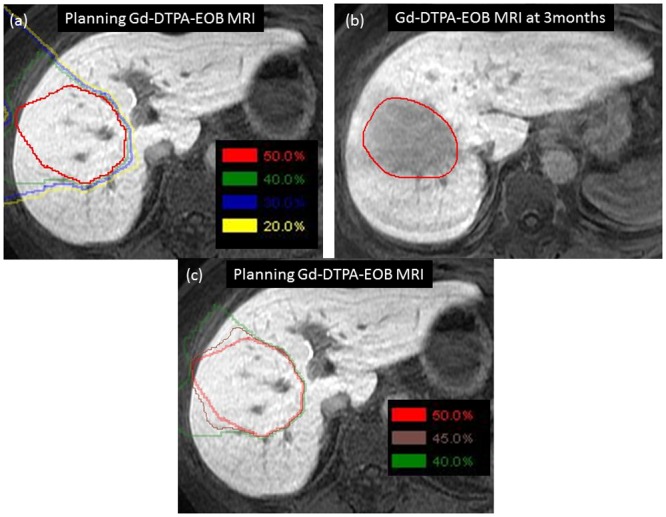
Method for defining the TD for FLR using hepatobiliary phase Gd-EOB-DTPA MRI at 3 months after PBT. The isodose lines were transferred onto each Gd-EOB-DTPA MRI. (a) The isodose lines are displayed as 50%, 40%, 30% and 20% lines. These dose lines were compared with the FLR contour on the planning MRI. (b) The medial dose line (45% line; brown) between the selected two doses (40% and 50% lines) was added. Finally, using these three isodose lines, the dose distribution contours that were most similar to the FLR contour were defined. In this case, TD was defined by the 45% dose line as 29.7 CGE. FLR contour, red (on the left side); 50% isodose line, red; 45% isodose line, brown; 40% isodose line, green; 30% isodose line, blue; 20% isodose line, yellow. Abbreviations; TD: Threshold dose; FLR: Focal liver reaction; Gd-EOB-DTPA MRI: Gadolinium ethoxybenzyl diethylenetriamine pentaacetic acid-enhanced magnetic resonance imaging; PBT: Proton beam therapy; CGE: Cobalt Gy equivalent.
